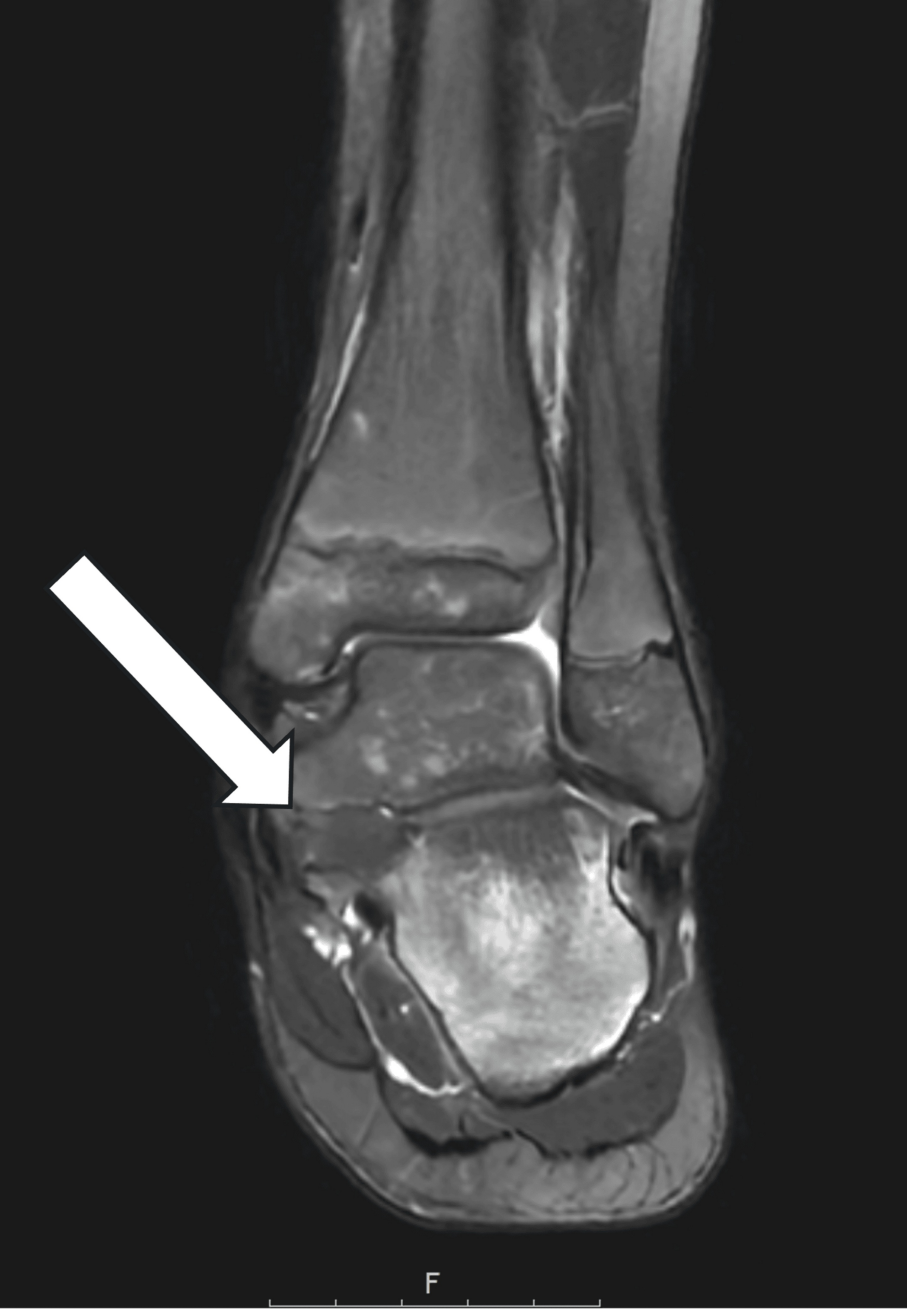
T2-weighted post-operative coronal MRI showing RegJoint™ marked with the white arrow.
